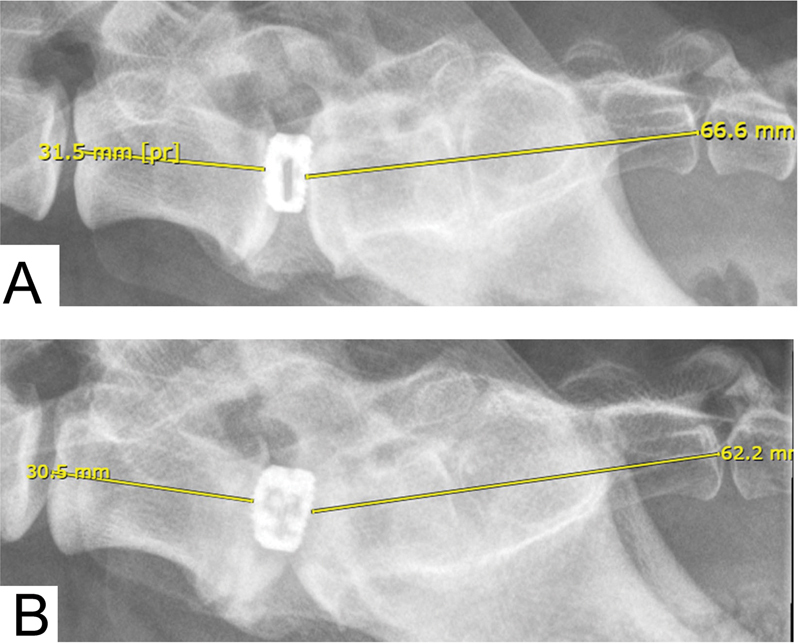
Measurement of vertebral body length of L7 and S1–S3 on the postoperative radiograph after placement of a stand-alone intervertebral spacer (
